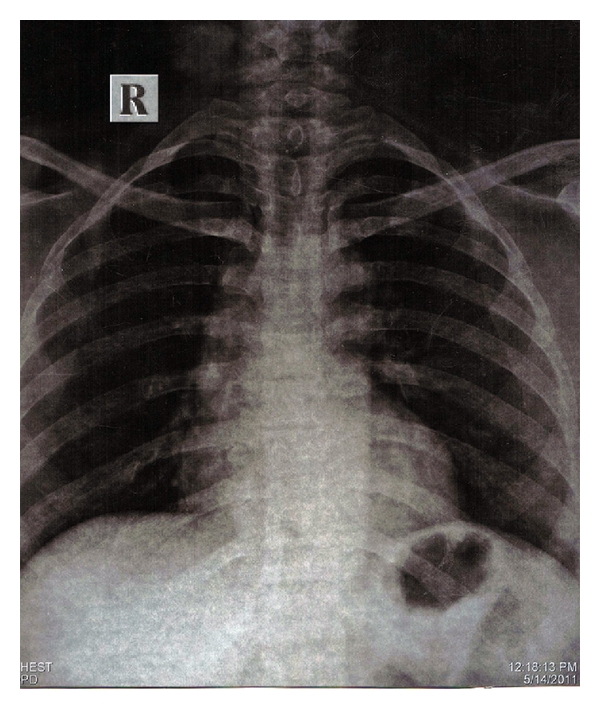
Chest radiograph after half an hour, showing no evidence of instrument.
